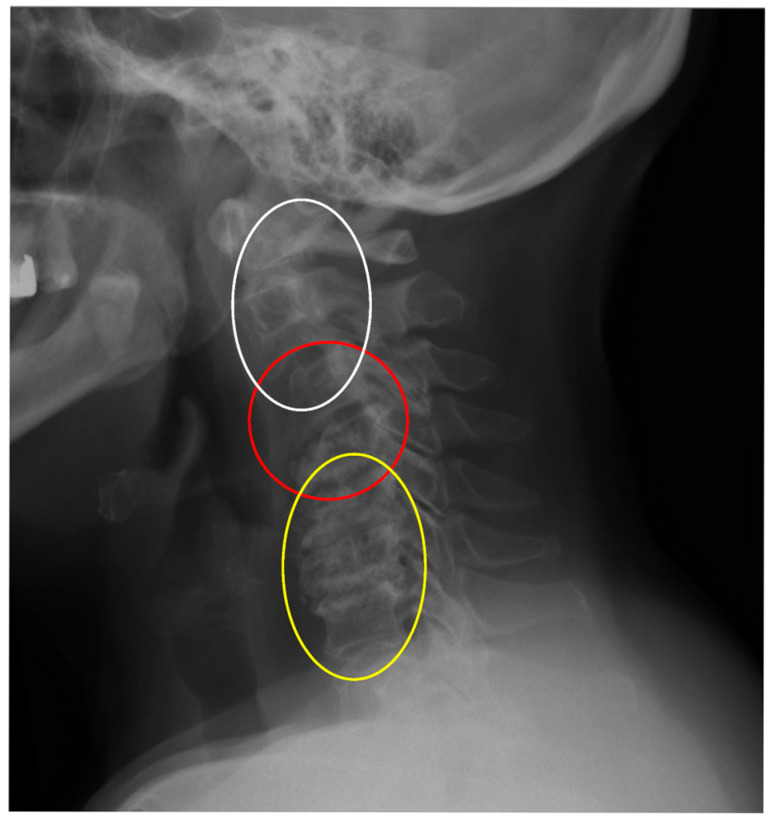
An X-ray of the cervical spine (lateral view) of a 67-year-old female patient with RA and SAS instability. C4–C5 instability (red). Cervical deformity with flat neck below the C4–C5 segment (yellow) and kyphotic deformity above the C4–C5 segment (white).
